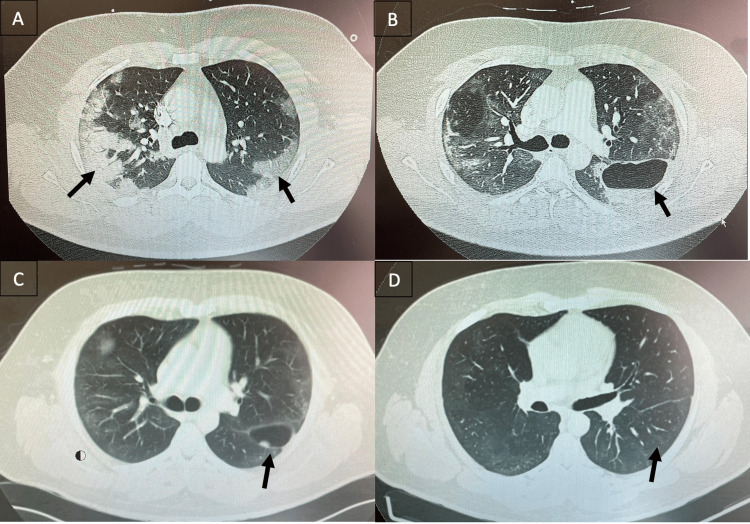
Serial axial chest CT images (lung window) on July 11 (A), August 7 (B), September 11 (C), and December 8 (D), 2021, showing the evolution of post-COVID lung changes. Initial scans demonstrate bilateral parenchymal abnormalities compatible with late-phase COVID-19 pneumonia, followed by the development of a large thick-walled cavitary lesion with an air-fluid level in the left lower lobe and subsequent near-complete radiological resolution with minimal residual changes.
